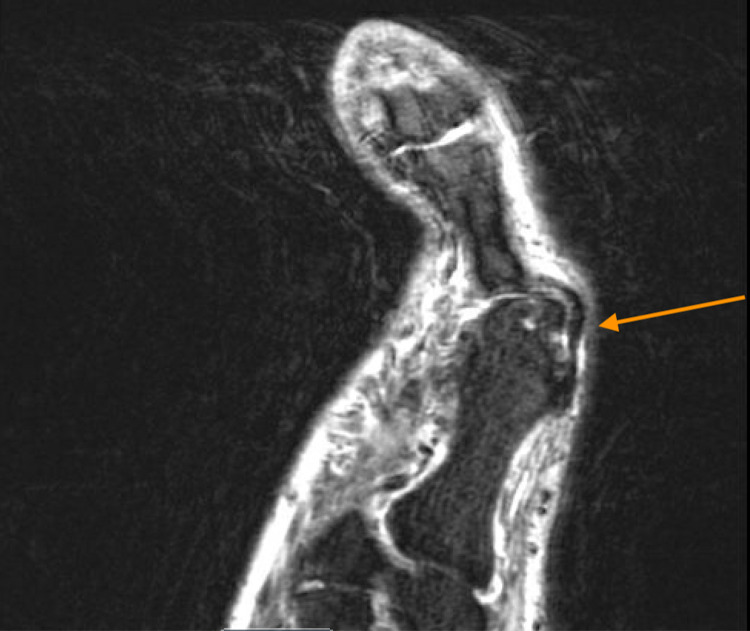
MRI foot demonstrating cellulitic inflammatory changes without osteomyelitis. Orange arrow points at the changes described above.
